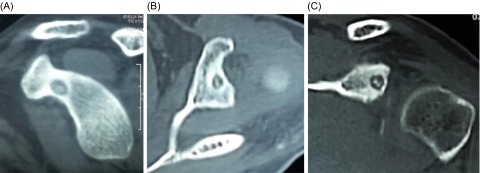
(A–C) CT scan in sagittal, transverse and coronal planes reveals the typical lesion of osteoid osteoma (OO) with a central nidus and surrounding sclerotic bone (to localize lesion pre-operative and plan excision).
